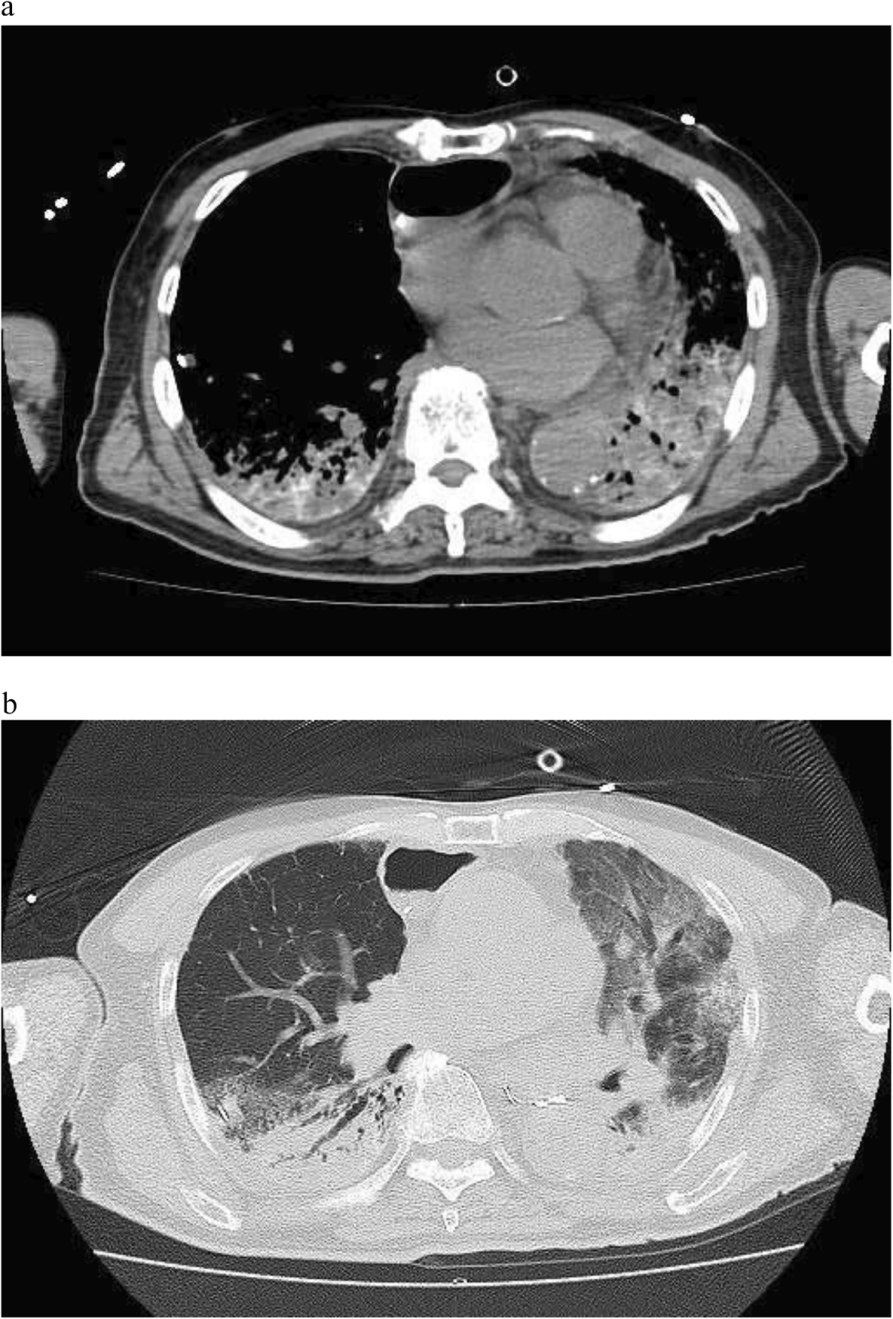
Computed tomography image showing high-density substance in the lung (a) and ground-glass opacity in bilateral lungs (b)
